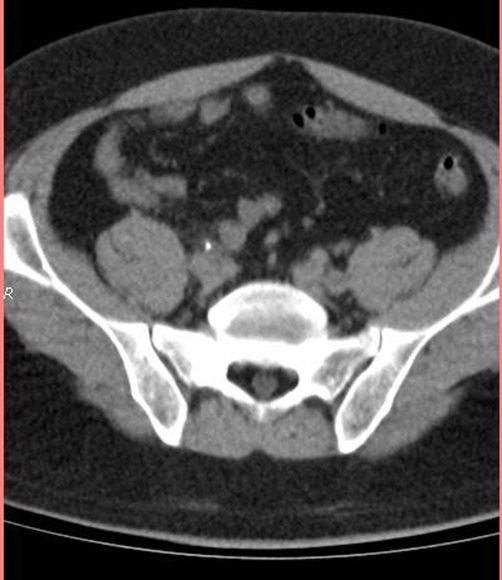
CT showing 8-mm hyperdense image in the right ureter topography.
